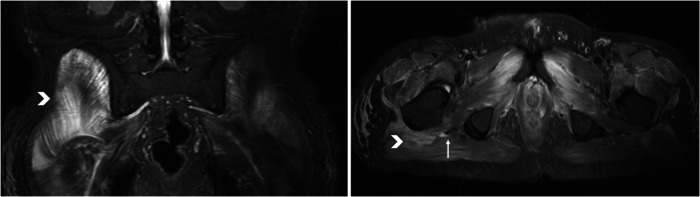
Embedded sciatic nerve (arrow) surrounded by muscular edema (arrowhead) on initial NHO on MRI of the pelvis (T2 sequence).
